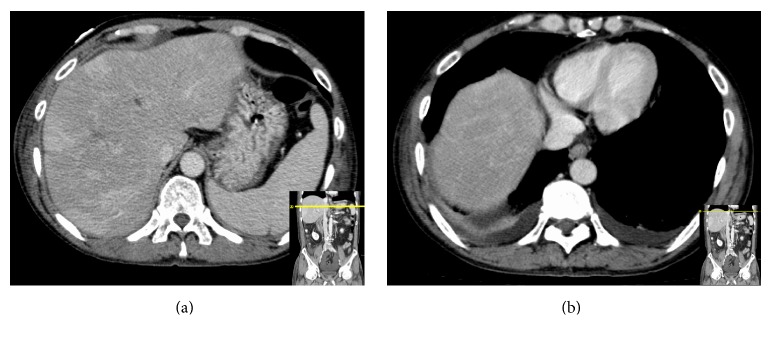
CT scan of the abdomen showing resolution of the portal venous gas.
